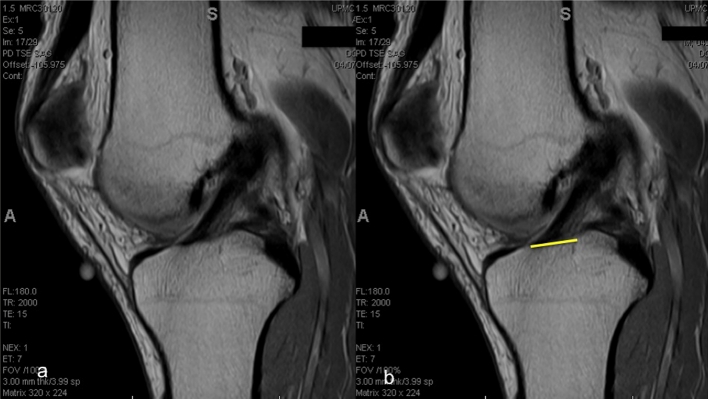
a Sagittal MRI cut best showing the ACL attachment to the tibia; b the most anterior and most posterior fibres attaching the tibia are connected by a line that represents the insertion site size
